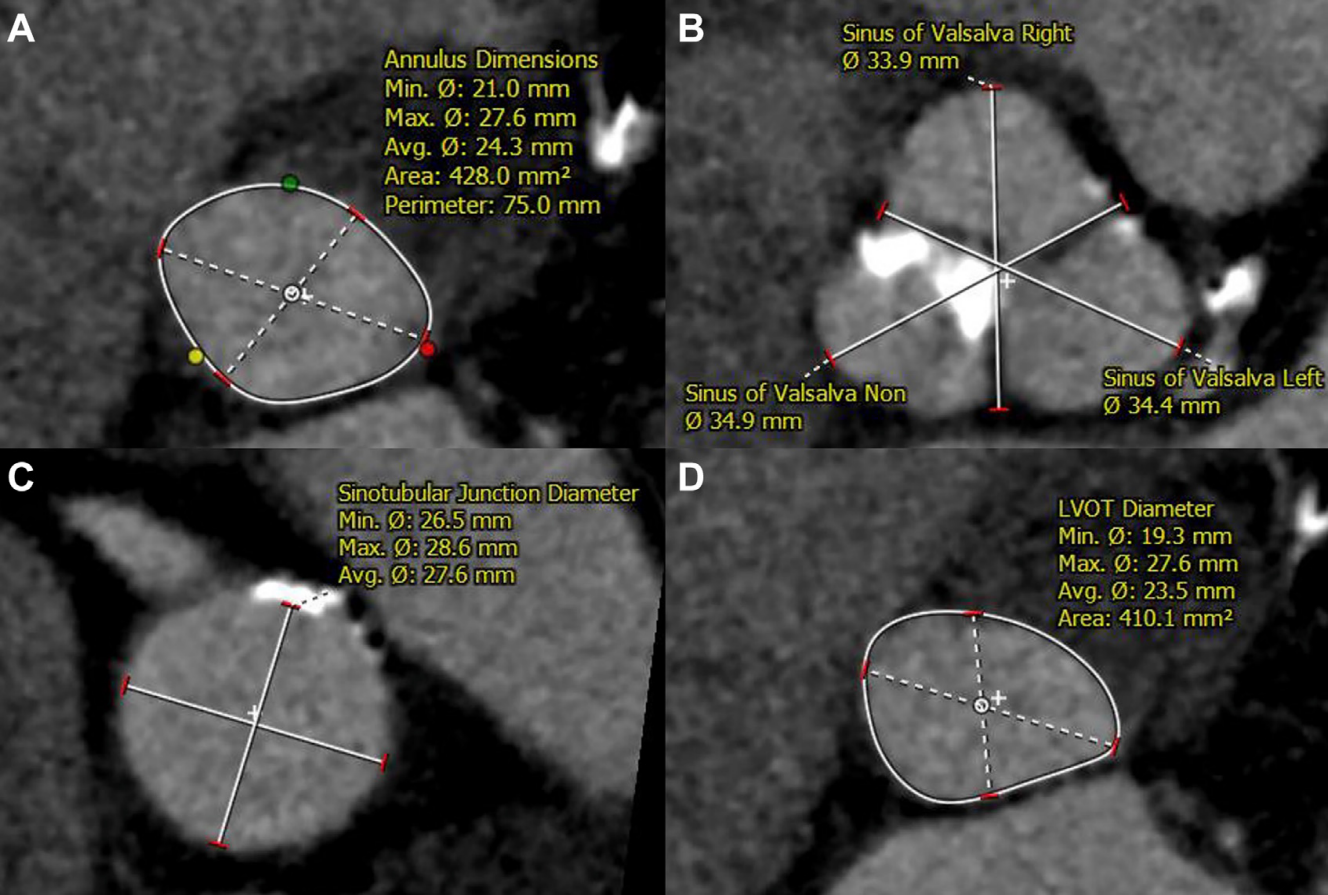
Computed Tomography Measurements of the Aortic Valve Complex Before Transcatheter Aortic Valve Replacement (A) Annulus. (B) Sinus of Valsalva. (C) Sinotubular junction. (D) Left ventricular outflow tract.
